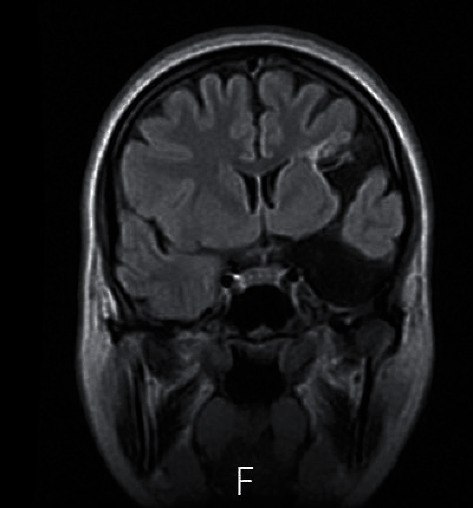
Coronal T1-weighted view showing the communication between the arachnoid cyst and the porencephalic cyst trough the Sylvian fissure, which makes this combination of conditions unique and characteristic.
